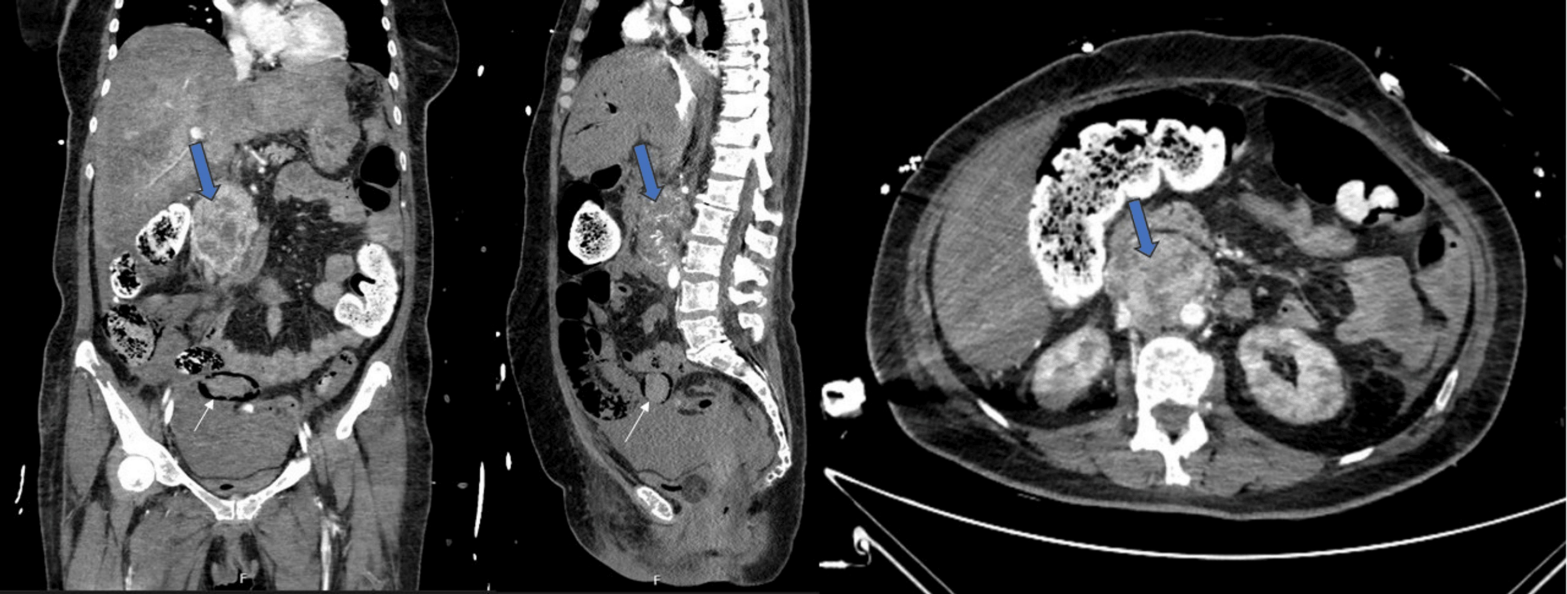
Coronal, sagittal, and axial CT images in the portal venous phase. Coronal (left), sagittal (center), and axial (right) portal venous phase CT images of the thoracoabdominopelvic region. The blue arrow identifies a heterogeneous retroperitoneal mass. The thin white arrow indicates intestinal pneumatosis.
